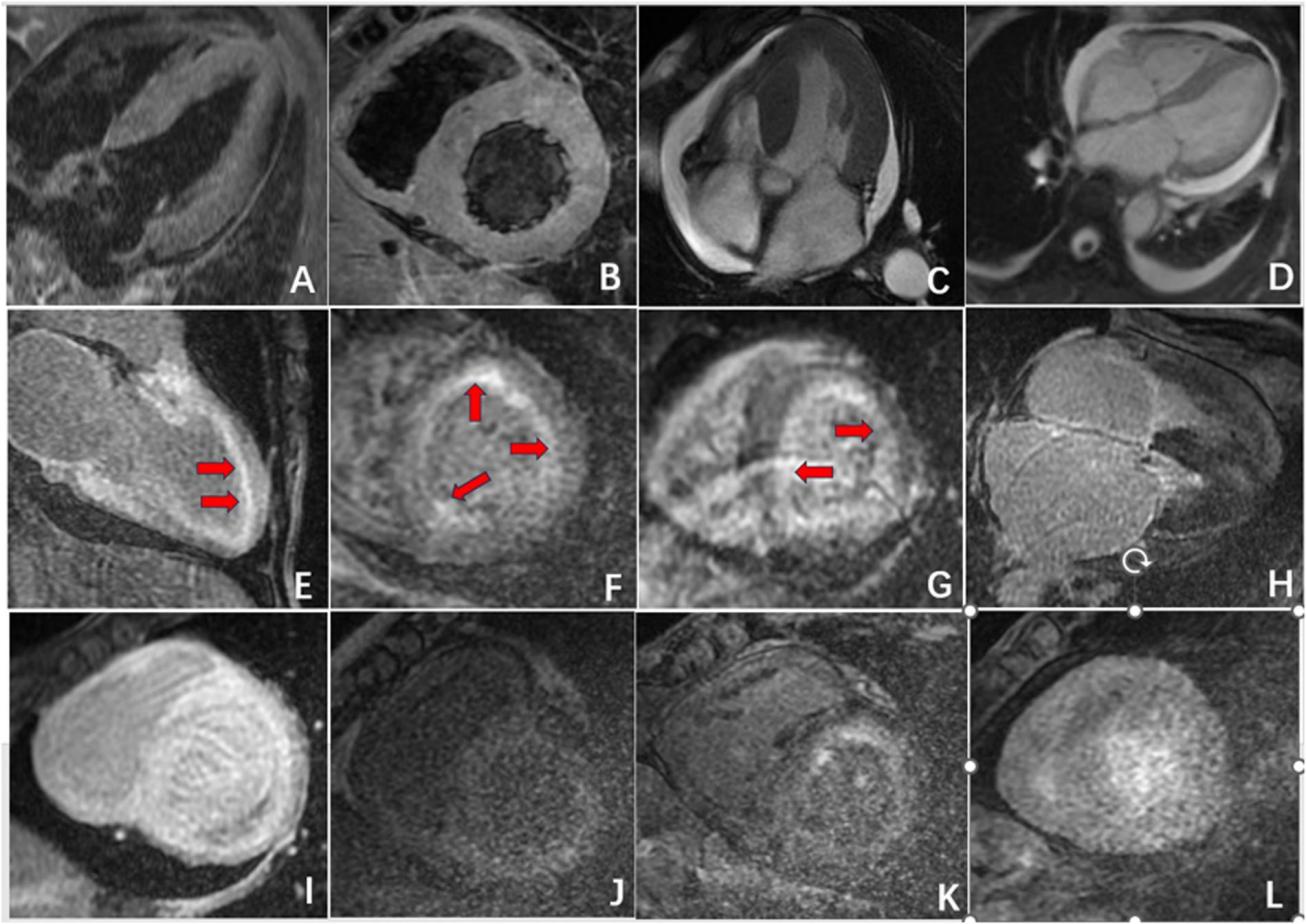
Radiographic findings of CA. (A,B) Symmetric myocardial hypertrophy; (C,D) pleural effusion and pericardial effusion; (E,F) subendocardial enhancement (red arrow); (G) transmural delayed enhancement pattern indicating diffuse myocardial infiltration; (H,I) delayed enhancement affected the left atrium, right atrium, and right ventricle; (J,L) “dark blood pool imaging” and “chaotic sign”.
